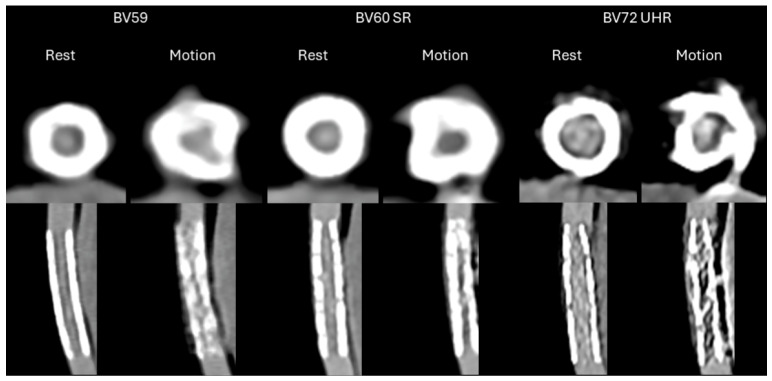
Longitudinal and axial slices of a stent (Braun Coroflex Blue (B. Braun SE, Melsungen, Germany)) at rest and in motion scanned with the EID CT, reconstructed with a Bv59 kernel, and scanned with a PCD CT in SR mode using the Bv60 kernel and using the Bv72 kernel in UHR mode. The window width was set to 1500 HU and the center to 300 HU.
